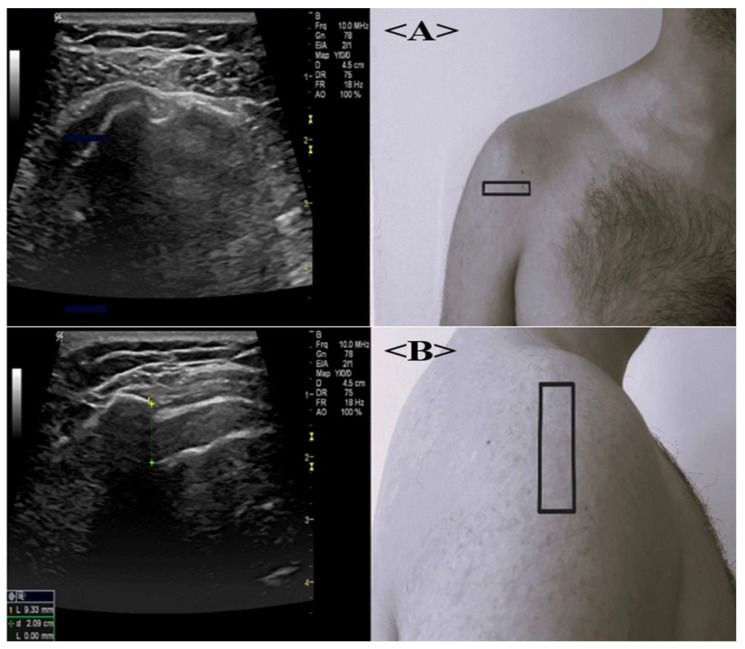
(A) Ultrasonographic image of the biceps tendon of a 56-year-old male patient with RA. (B) Ultrasonographic image of the acromiohumeral distance (AHD) measurement. The black rectangular boxes on the clinical photographs indicate the approximate transducer placement and anatomical region evaluated during ultrasonographic examination. The colored line and caliper markers on the ultrasound image in panel (B) represent the AHD measurement.
